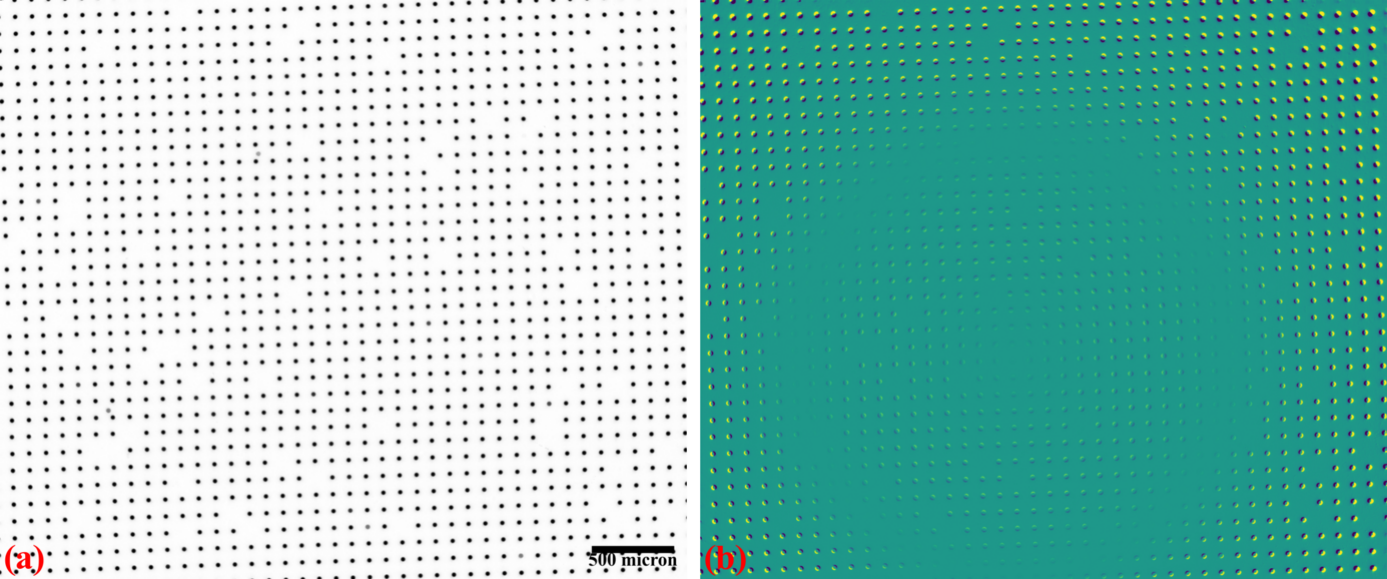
(*a*) X-ray dot-target image, (*b*) difference between the images before and after distortion correction.
